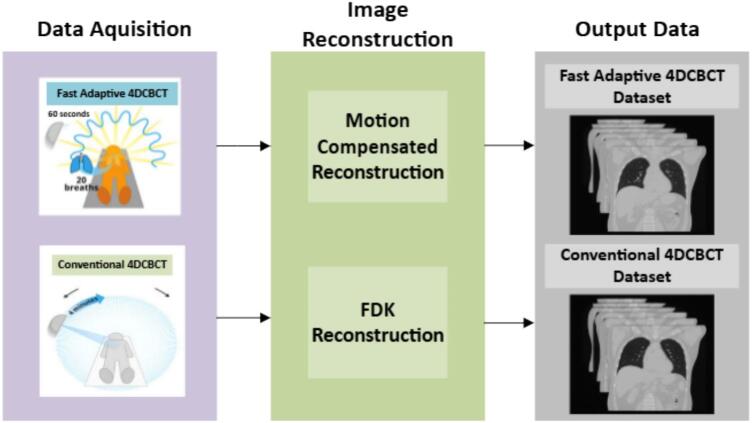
Overview of 4DCBCT datasets: fast adaptive and conventional acquisition protocols. FDK, Feldkamp–Davis–Kress reconstruction algorithm.
